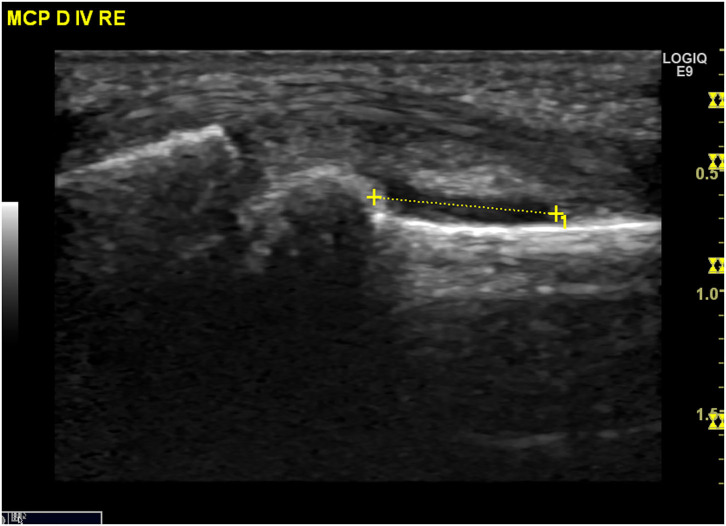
Ultrasound assessment of synovitis metacarpophalangeal joint digit IV (MCP D IV).
